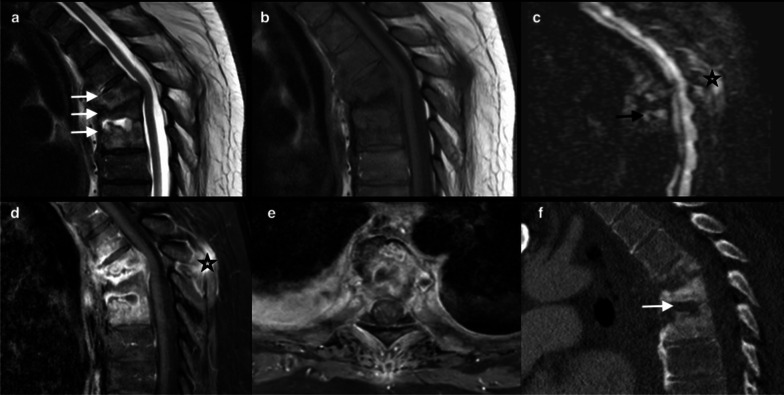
72-year old male with amyotrophic lateral sclerosis presenting with subacute back pain, fever and inflammation in laboratory findings. Sagittal T2 (a), T1 (b) WI, DWI (c) and T1 after contrast on sagittal (d) and axial planes (e) show multiple contiguous erosive disco-vertebral disease on dorsal spine with local deformity and advanced destruction (arrow). No diffusion restriction was noticed on DWI (black arrow). Note anterior and posterior involvement (star). Reformatted sagittal CT (f) shows bone sclerosis and subtle vacuum phenomena in the disc (arrow). R1 Point 11The final diagnosis was Charcot spine
